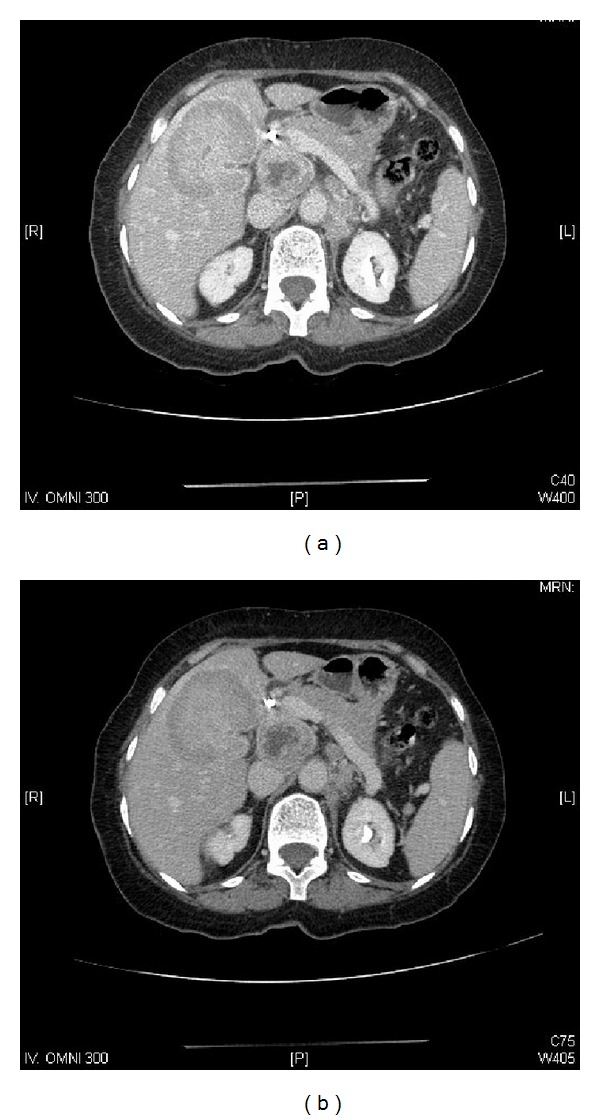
Prechemotherapy abdominal computed tomography (CT) showing (a) a large 7.9 × 5.1 cm heterogeneous mass involving the gallbladder and the adjacent liver and (b) enlarged portocaval and para-aortic lymph nodes.
